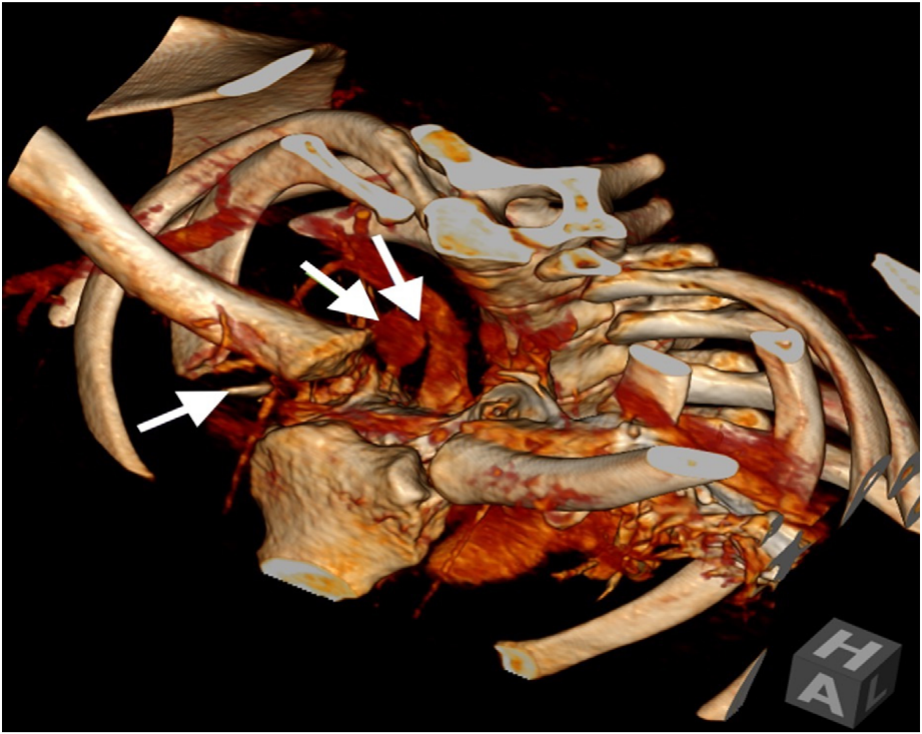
Three-dimensional reconstruction of the thoracic inlet using the computed tomography angiogram of the head and neck demonstrates fracture of the right clavicular head with an anterior fracture fragment posterior displacement of the clavicle with abutment of the pseudoaneurysm/contained rupture (arrows). The connecting neck extending from the brachiocephalic artery is also depicted.
